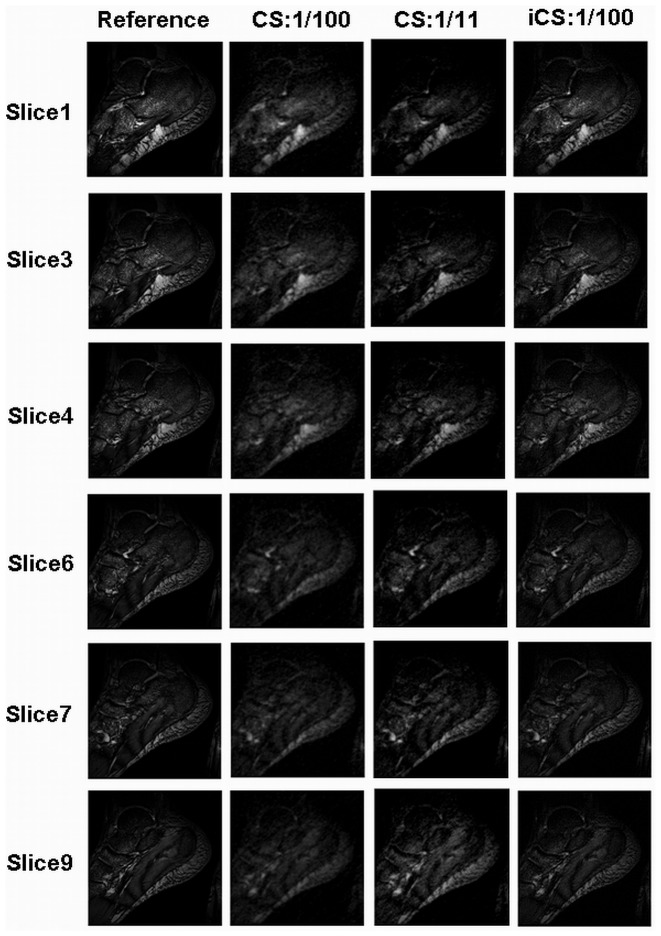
In-vivo MR images of human feet in sagittal plane. Each slice (i.e., slice #1, #3, #4, #6, #7, and #9) was reconstructed using the three methods: conventional full k-space reconstruction (which served as the reference in the 1^st^ column), conventional Compressed Sensing at undersampling rate of 1/100 (in the 2^nd^ column), conventional Compressed Sensing at undersampling rate of 1/11 (in the 3^rd^ column), and the proposed interpolated CS methods at the undersampling rate 1/100 (in the 4^th^ column). In this comparison study, image quality improvement of the iCS reconstruction method is observed compared with the CS method, much image details being recovered.
